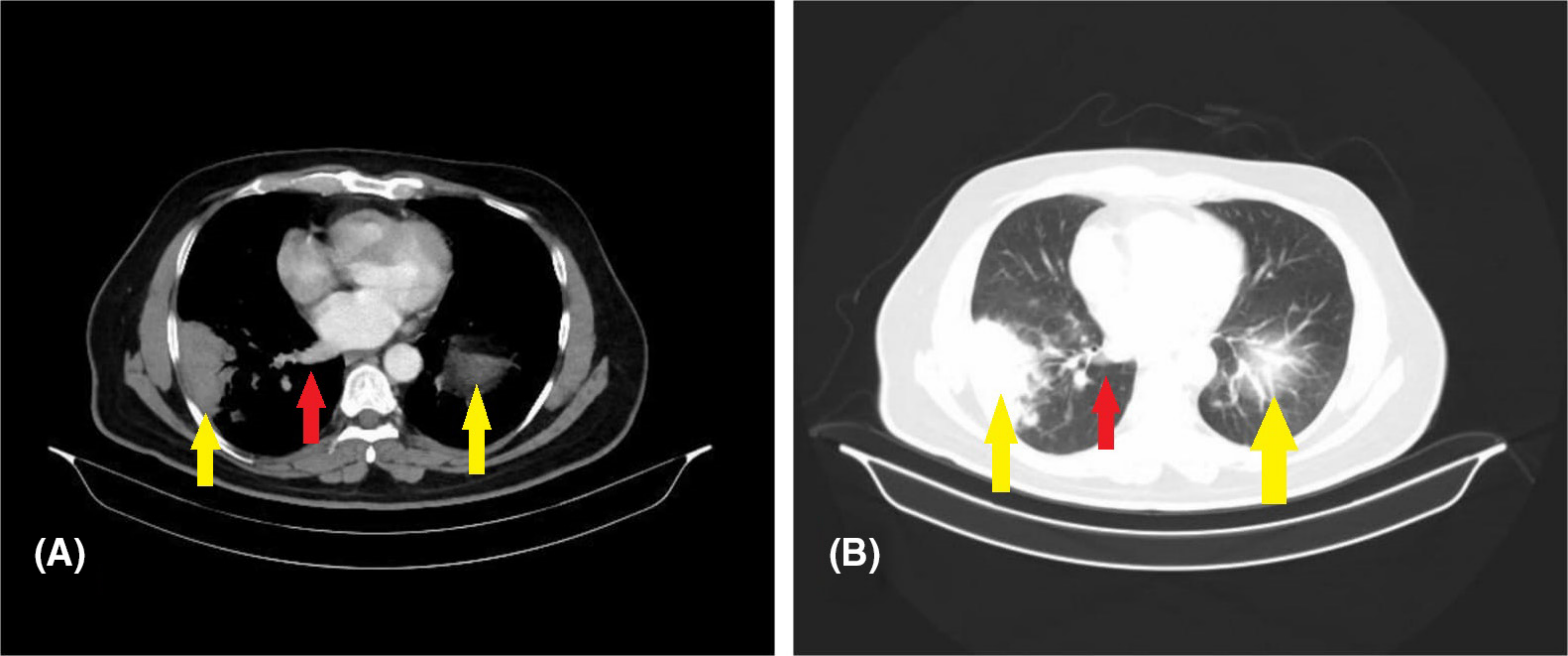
(A) CT scan of the chest with contrast and (B) CT scan of the chest without contrast, showing soft tissue density in the lower base of the right lung (yellow arrow), accompanied by an enlargement of the right hilar lymph node (red arrow).
